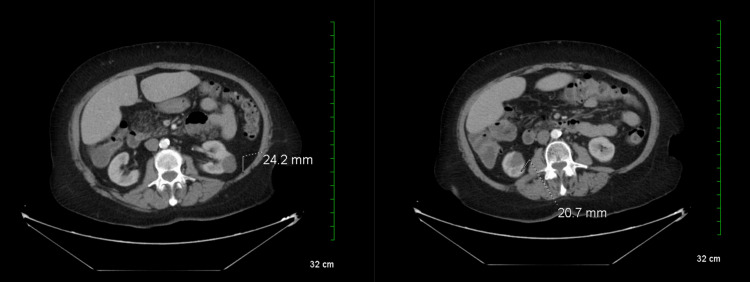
CT scan (abdomen) CT scan images reveal bilateral renal cysts. The cyst on the left measures 2.4 cm, while the cyst on the right measures 2.1 cm. CT: Computed tomography
